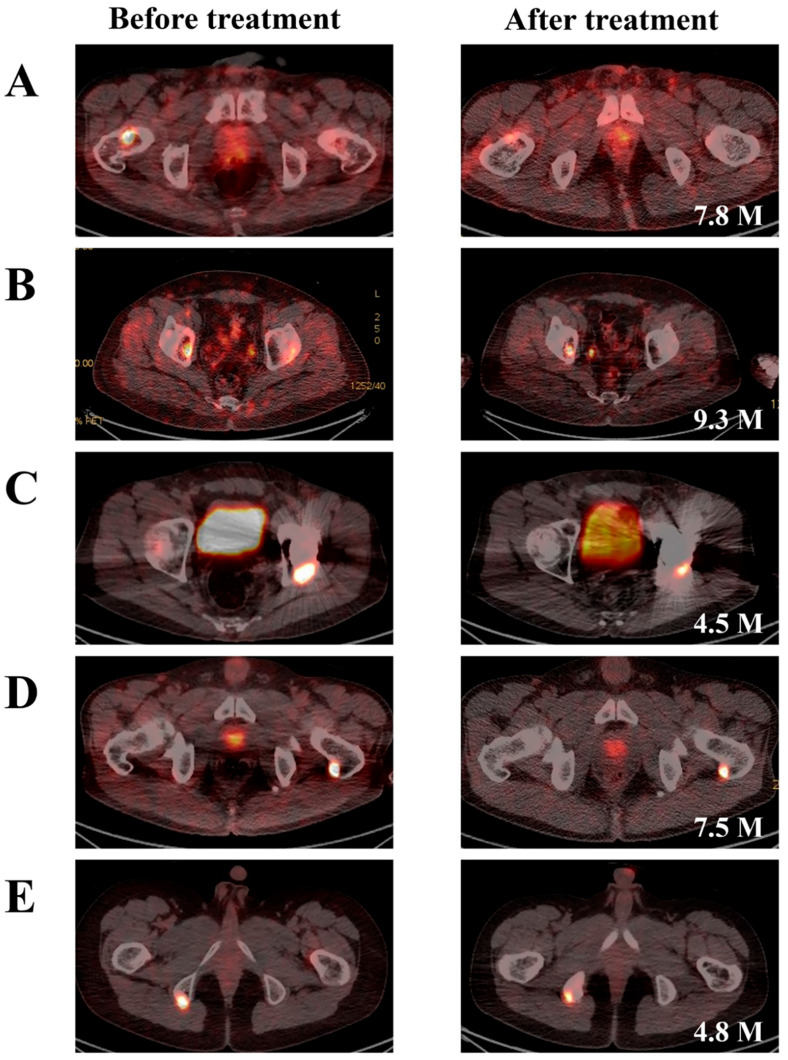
Detection of residual PMTs after first treatment using ^68^Ga-DOTATOC PET/CT. If a patient’s symptoms did not improve, a second ^68^Ga-DOTATOC PET/CT was performed, and if any residual tumor was detected, the treatment was ultimately judged to have failed. The white text in the lower right corner of the right panel indicates the period (in months) after the first treatment. (A) patient No. 2; (B) patient No. 6; (C) patient No. 9; (D) patient No. 12; and (E) patient No. 13.
